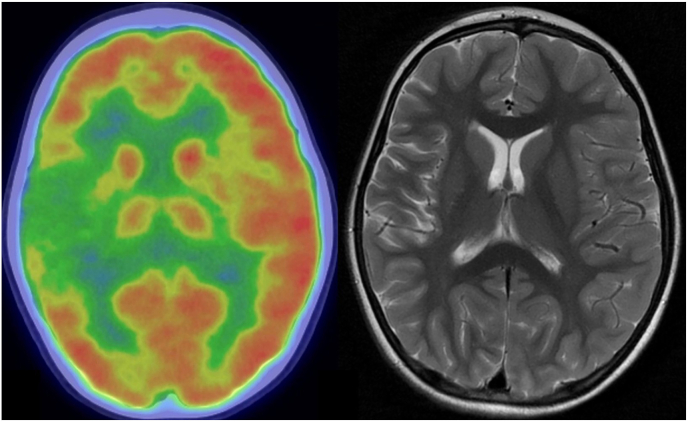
Rasmussen encephalitis: 13yrs old male, epilepsia partialis continua left arm and progressive hemiparesis. FDG-PET/CT transversal (left) and T2 weighted MRI transversal (right): Hypometabolism and slight hemiatrophy of the right hemisphere.
